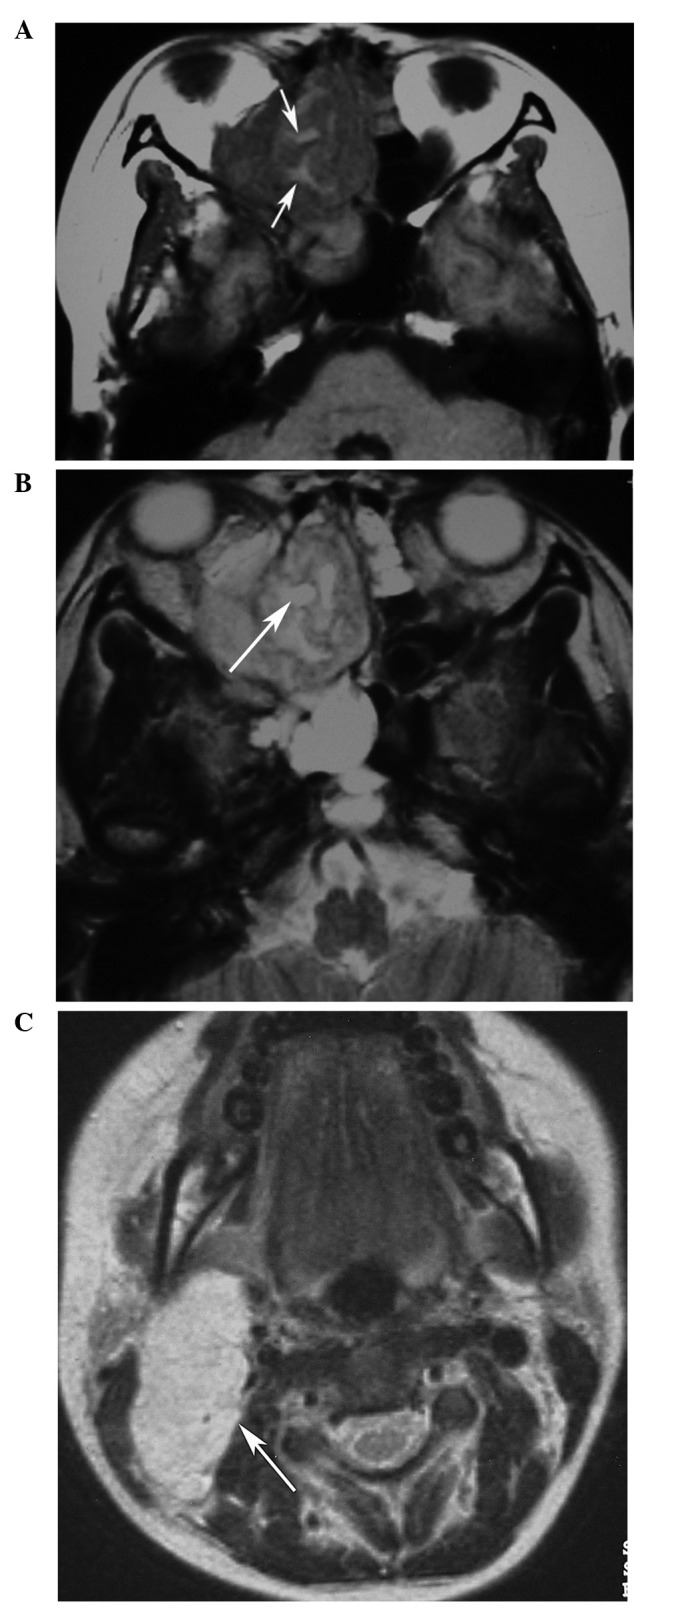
Case 3, a 17-year-old female with embryonal rhabdomyosarcoma in the ethmoid sinus. (A) Axial T1-weighted imaging (WI) shows an isointense mass originating from the right ethmoid sinus with multiple hyperintense signals (arrow). (B) Axial T2WI shows the mass with heterogeneous hyperintensity (arrow) and (C) axial T2WI demonstrates enlarged lymph nodes (arrow) involving the right side of the neck.
